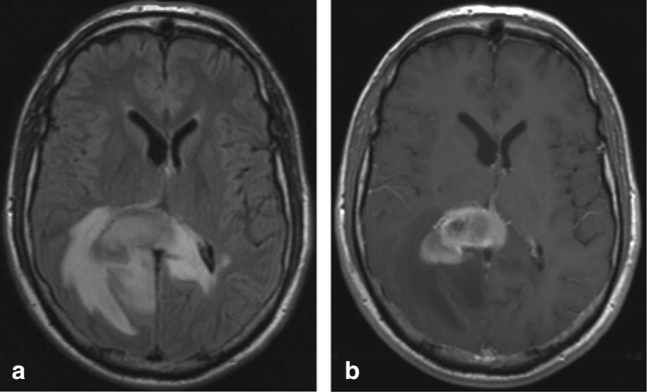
Forty-seven-year-old man with biopsy proven B cell lymphoma. Axial FLAIR (a) shows a space occupying lesion with a slightly hyperintense center and a hypointense rim in the splenium, which enhances vividly after contrast (axial T1 with gadolinium (b)
